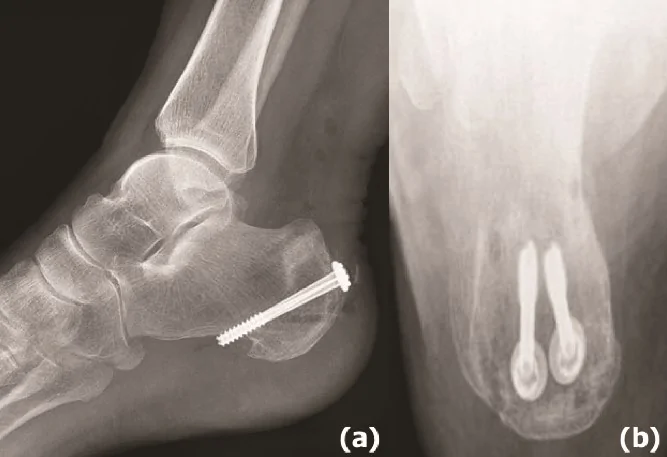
Postoperative radiographs. (a) Sagittal image, (b) axial image.
